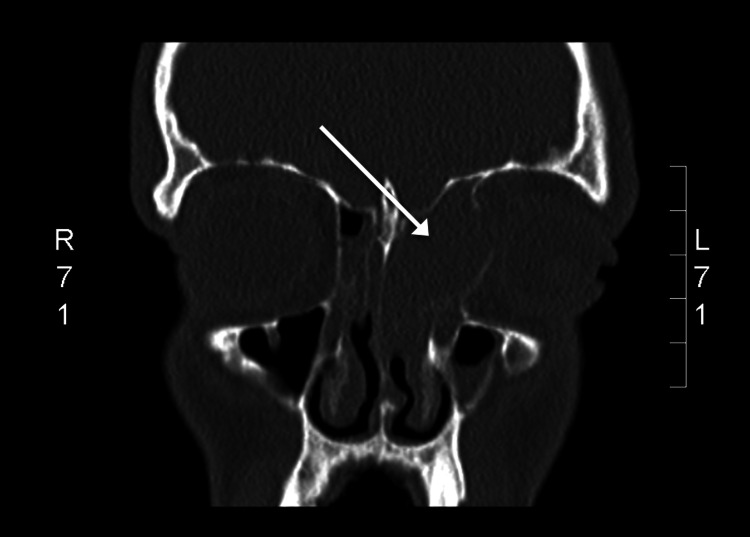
Coronal CT sinus showing near complete opacification of left anterior ethmoid sinus with obvious bony destruction. The mass effect on the left orbit is obvious with deviation to the left and the globe pushed anteriorly.
